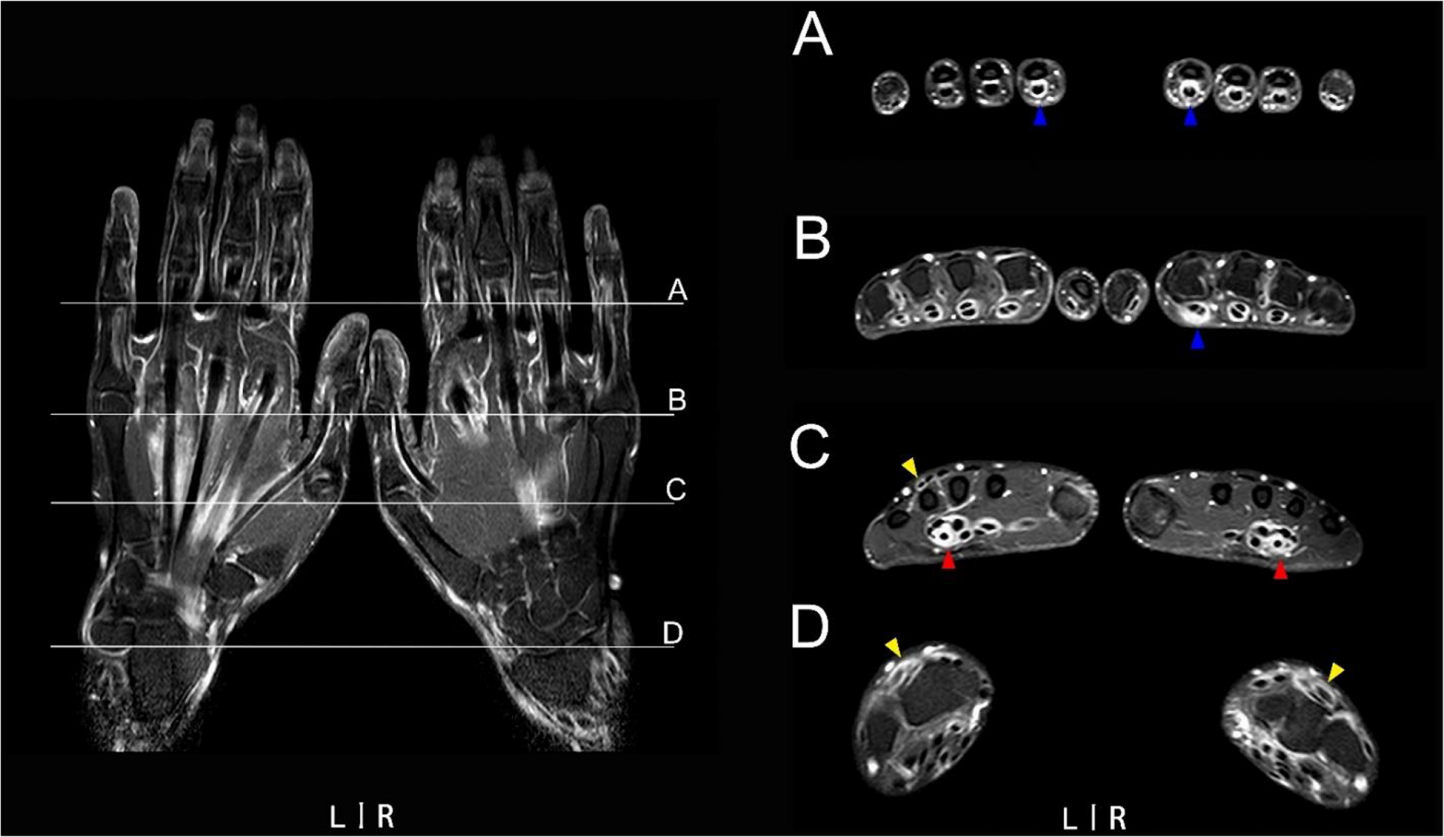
A typical MRI image of tenosynovitis. The patient was a 29-year-old female with 11 months of RA disease duration and DAS28-CRP of 4.52, and she never took any disease-modifying anti-rheumatic drugs or glucocorticoid. Left, coronal spin echo fat-suppressed T1-weighted imaging; right (A–D), axial spin echo fat-suppressed T1-weighted imaging. (A) Proximal interphalangeal joints 2~5; (B) interphalangeal joint of thumb and metacarpophalangeal joints 2~5; (C) metacarpophalangeal joint 1 and the distal part of wrist; and (D) the proximal part of wrist. Blue arrows, flexor tenosynovitis of digit in PIPJ or MCPJ; red arrows, tenosynovitis in bilateral wrist flexor compartments 2; yellow arrows, tenosynovitis in bilateral wrist extensor compartments IV
